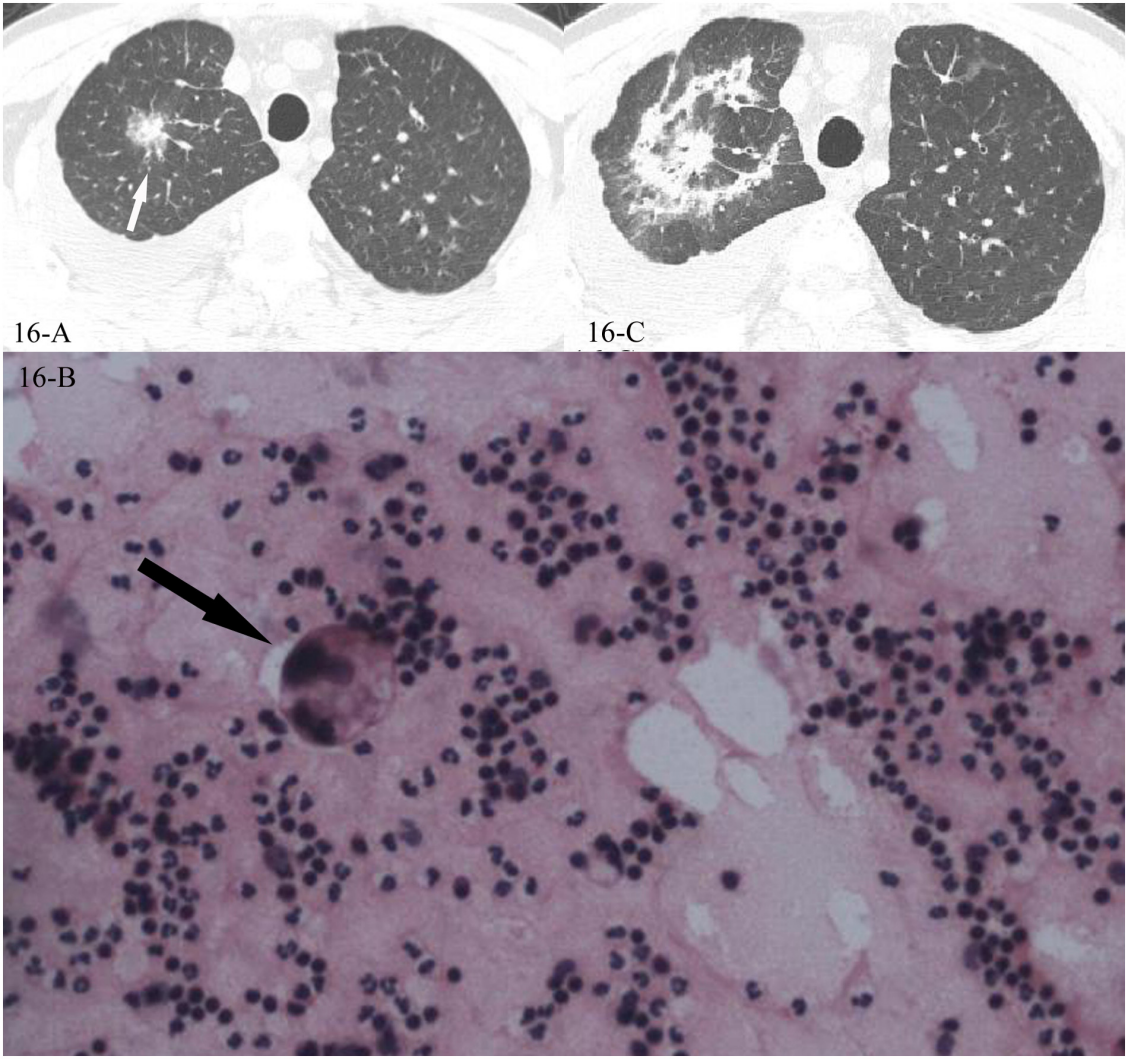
A, B, C. A 42-year-old male diagnosed with adenocarcinoma by pleural effusion, the HRCT showed a mass in the right upper lobe(16-A) and adenocarcinoma cells were found in the pleural effusion smear with the background the lymphocytes(16-B, black arrow, 200x magnification), no chemotherapy but just pleural fluid drainage was performed, and 6 weeks later the follow-up CT scan showed reversed halo sign(16-C).
